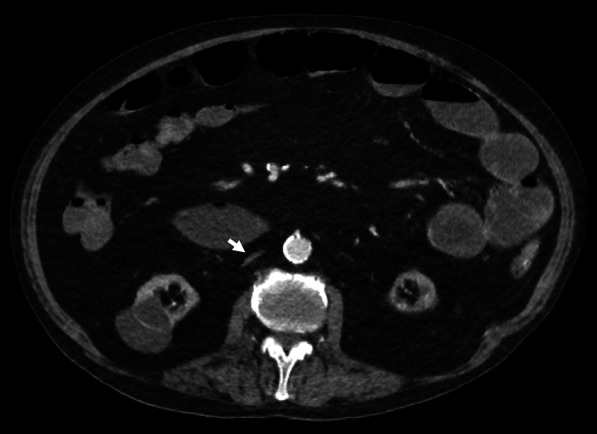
Slit-like IVC from hypovolaemia. Axial-enhanced CT image demonstrates a slit-like IVC or ‘flat cava’ sign (arrow), consistent with the patient’s clinically evident hypovolaemia following surgery
